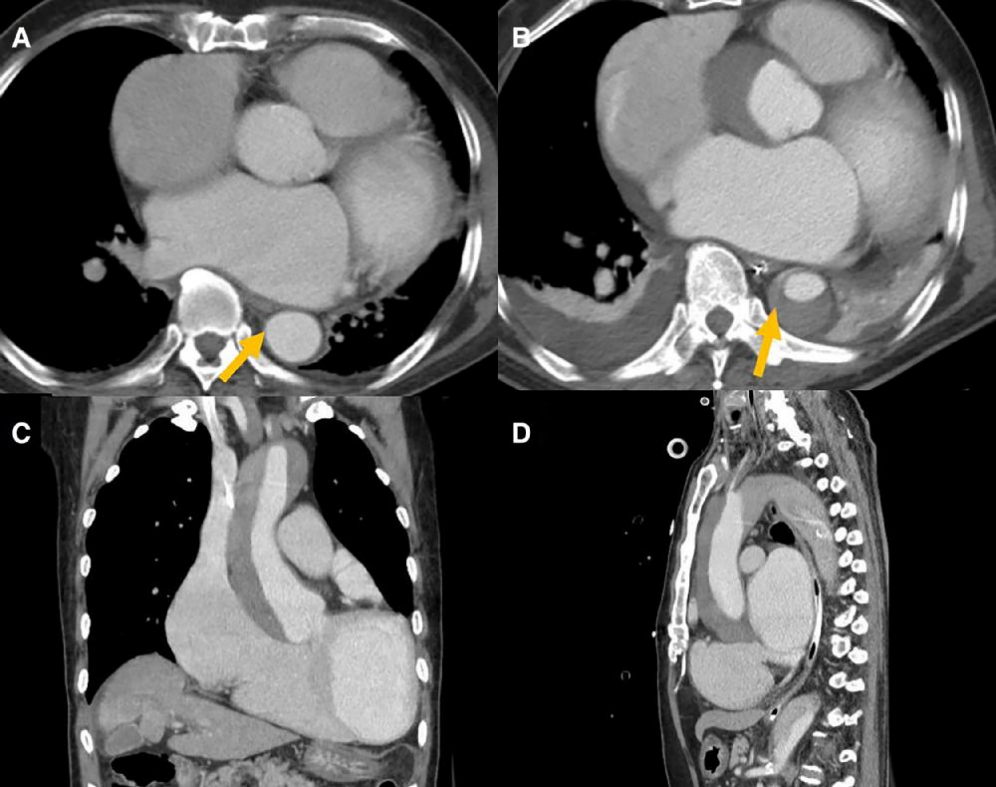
Computed tomography (CT) images before and after the transoesophageal echocardiogram (TEE) procedure. (*A*) The pre-procedural CT at the same level of suspected intimal flap, no signs of the dissection was noticed. (*B*) The axial view of the post-procedural CT showed the location of the suspected intimal flap, where contrast enhancement in the false lumen can be noticed (arrow). (*C*) Coronal view of the type A aortic dissection. (*D*) Sagittal view of the type A aortic dissection.
